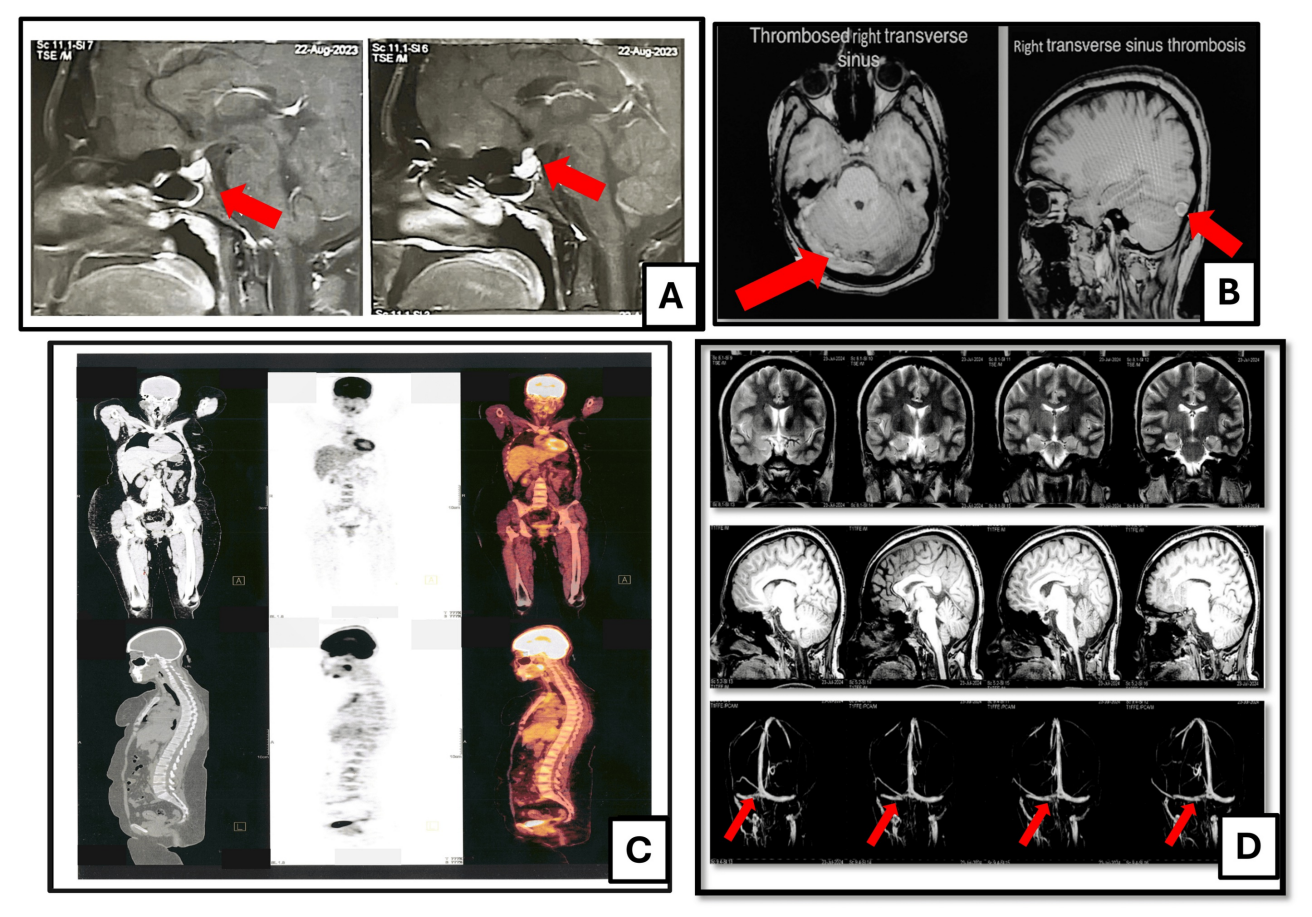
Radiological assessment of case 1. (A) MRI of the sella showing bulky posterior pituitary gland harboring a 7 mm hypo-enhancing lesion (red arrow), associated with thickened pituitary infundibulum reaching 6 mm in anteroposterior (AP), sagittal section (SS), and craniocaudal (CC) dimensions and 5 mm in SS dimension, representing suprasellar extension. (B) The right transverse and sigmoid sinuses show a loss of normal signal and no flow on post-contrast sequences (red arrows). (C) PET/CT scan showing prominent palatine tonsil, adenoid, and thymic tissue showing fluorodeoxyglucose uptake. (D) MRI of the brain (upper and middle rows) with magnetic resonance venography (MRV) (lower row) showing bilaterally distal transverse sinus stenosis (red arrows), focal on the right side and long segmental on the left side, followed by reconstitution at sigmoid venous sinus.
